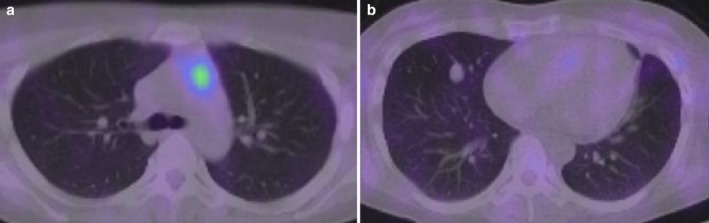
Somatostatin receptor scintigraphy showed abnormal accumulation in the anterior mediastinal mass (a) and pulmonary lesions (b).
